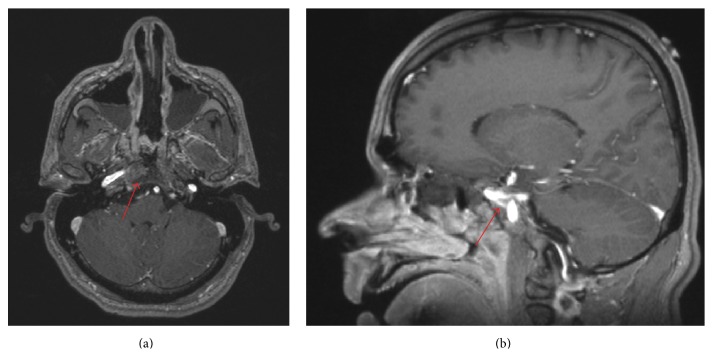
Postoperative magnetic resonance imaging, with contrast, axial (a) and sagittal (b) sections, demonstrating a small amount of residual tumor.
